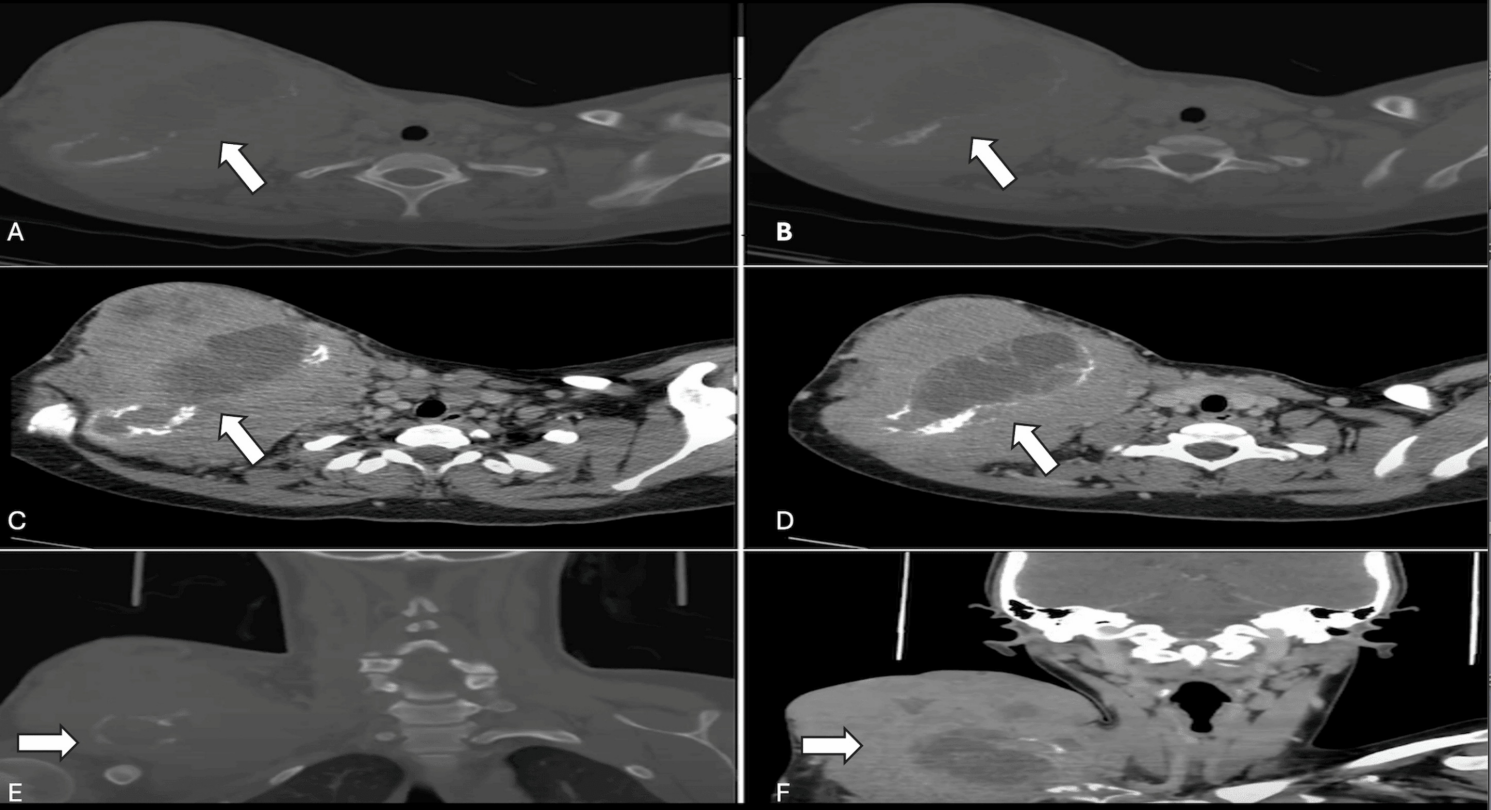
CT scan of the right clavicle before chemotherapy The axial bone view cuts (A and B) show the extensive destruction of the clavicle by the lytic lesion (white arrow). The axial soft tissue view cuts (C and D) show the soft tissue component of the lytic lesion along with the mass effect on the nearby vessels and structures (white arrow). The coronal views (E and F) show a large destructive lytic lesion emerging from the mid-clavicular lesion (white arrow). The images were taken from the patient's electronic records after securing consent from the patient for the publication, reproduction, broadcast, and other use of imaging and photos.
